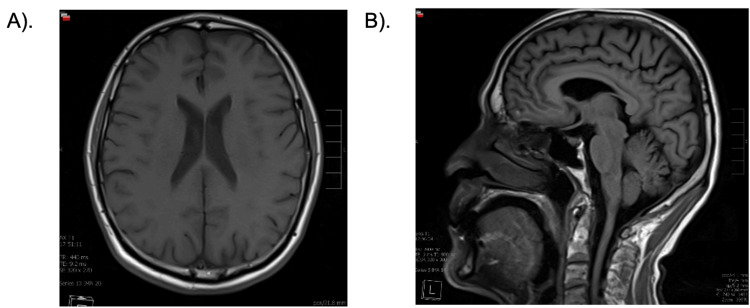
Magnetic resonance imaging of the brain with and without contrast in the transverse (A) and sagittal views (B).
